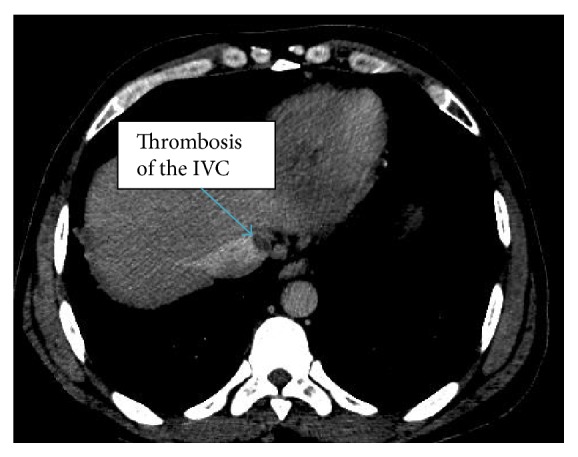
CT scan showing thrombosis of the hepatic portion of the IVC.
